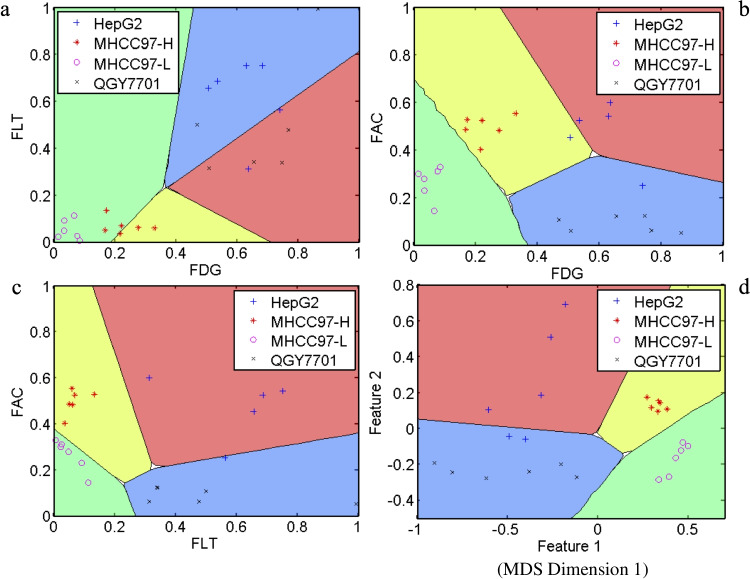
The testing dataset using Fisher’s classifier. a Testing dataset on 2 features ([^18^F]FDG + [^18^F]FLT), error rate 0.362. b Testing dataset on 2 features ([^18^F]FDG + [^18^F]ACE), error rate 0.085. c Testing dataset on 2 features ([^18^F]ACE + [^18^F]FLT), error rate 0.116. d Testing dataset on 3 features ([^18^F]FDG + [^18^F]ACE + [^18^F]FLT), error rate 0.074. Feature 1 (X-axis) represents the dimension with the greatest variation in distances between data points, and Feature 2 (Y-axis) represents the dimension with the second-greatest variation
